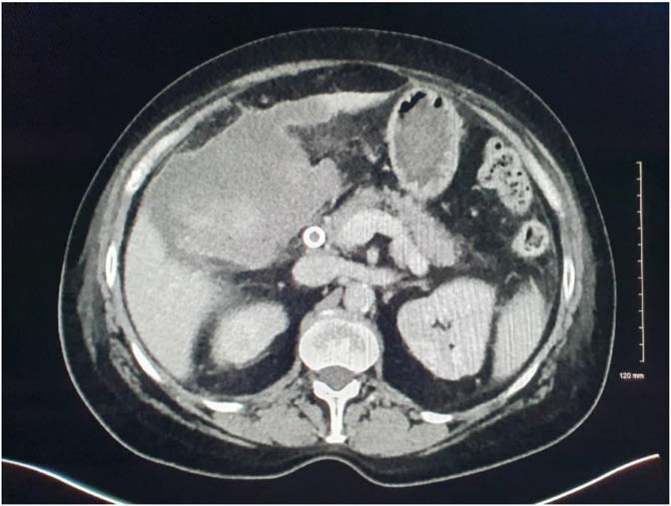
CT demonstrating superinfection of the gallbladder fossa haematoma and abscess formation.
